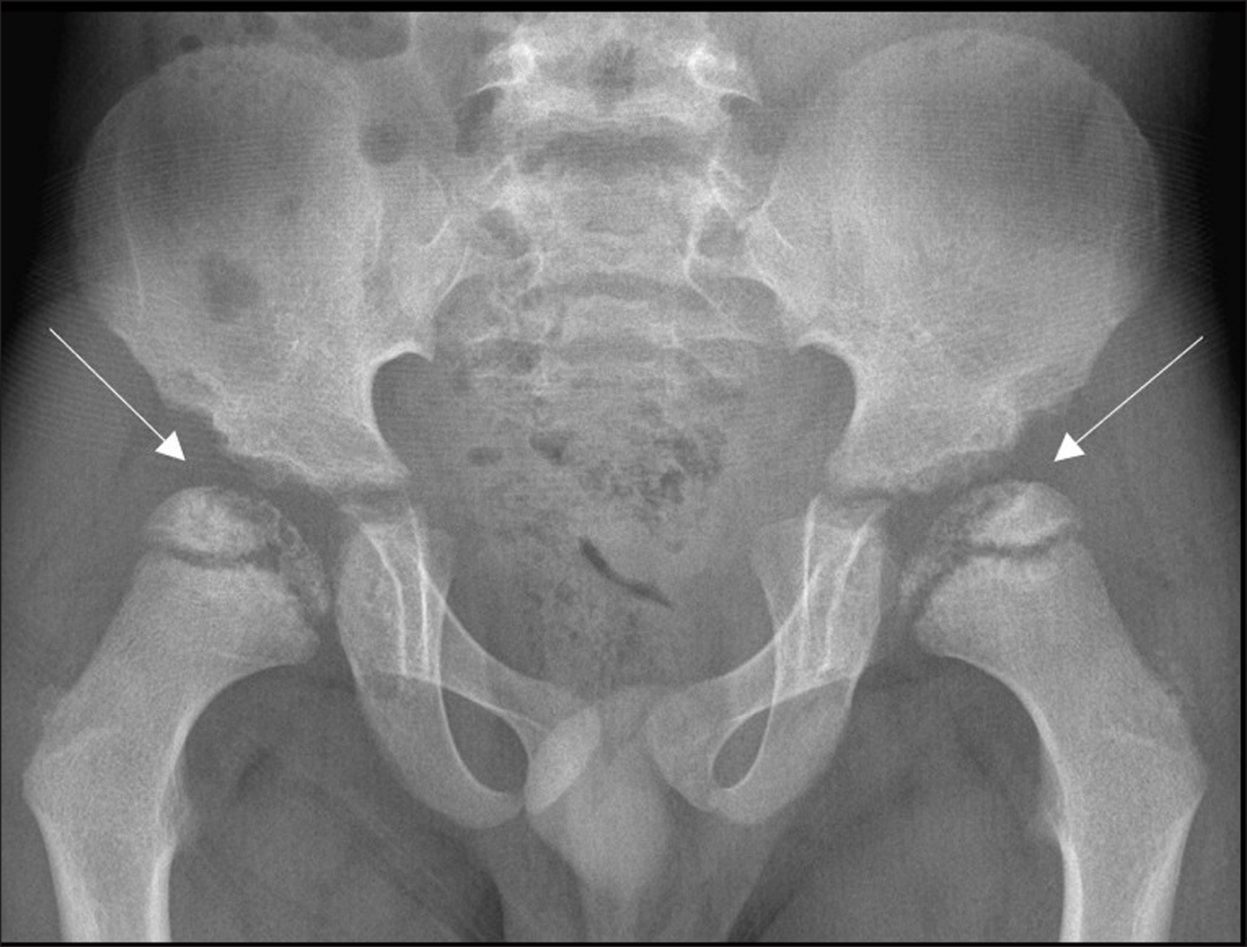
Based on the combination of clinical and imaging features a storage disease was suspected and additional examinations were performed. A radiograph of the hand showed a V-shaped deformity of the wrist, hypoplastic morphology of the proximal metacarpals (*Figure 3*, white arrows) and a delay in carpal ossification (*Figure 3*, asterix). The skeletal manifestations were suggestive of dysostosis multiplex congenita. MRI of the spine showed no spinal stenosis and no cord anomaly and confirmed the vertebrae hypoplasia with posterior scalloping (*Figure 1B*, white arrows). Urinary examination exposed high dermatan sulphate and chondroitin sulphate levels in keeping with mucopolysaccharidosis VI. The diagnosis of mucopolysaccharidosis VI was confirmed by enzymatic analysis of arylsulphatase B in leucocytes and homozygosity of the c.937C>G mutation (exon 5 ARSB gene). Both parents were carriers of the same mutation although consanguinity was not known.
